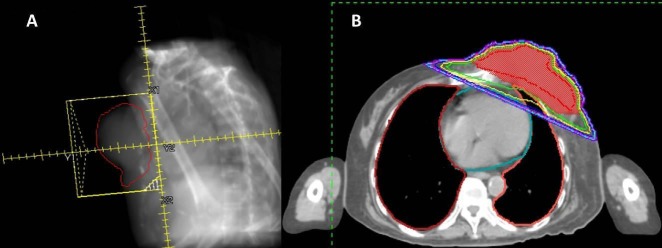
(A) Beam’s eye view (BEV) digitally reconstructed radiograph (DRR) of opposed tangential 6 megavoltage (MV) photon radiation fields. (B) Representative cross-sectional computed tomography (CT) planning image showing radiation isodose coverage of the tumor.
